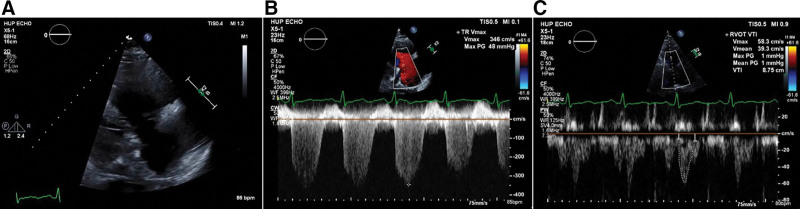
A–C, Transthoracic echocardiography 12 mo before liver transplantation. A moderate-to-severely dilated right ventricle with moderately decreased function, moderate-to-severe tricuspid regurgitation with pulmonary artery systolic pressure measuring 87 mm Hg. Mid-systolic notching of the right ventricular outflow track velocity time integral is present.
